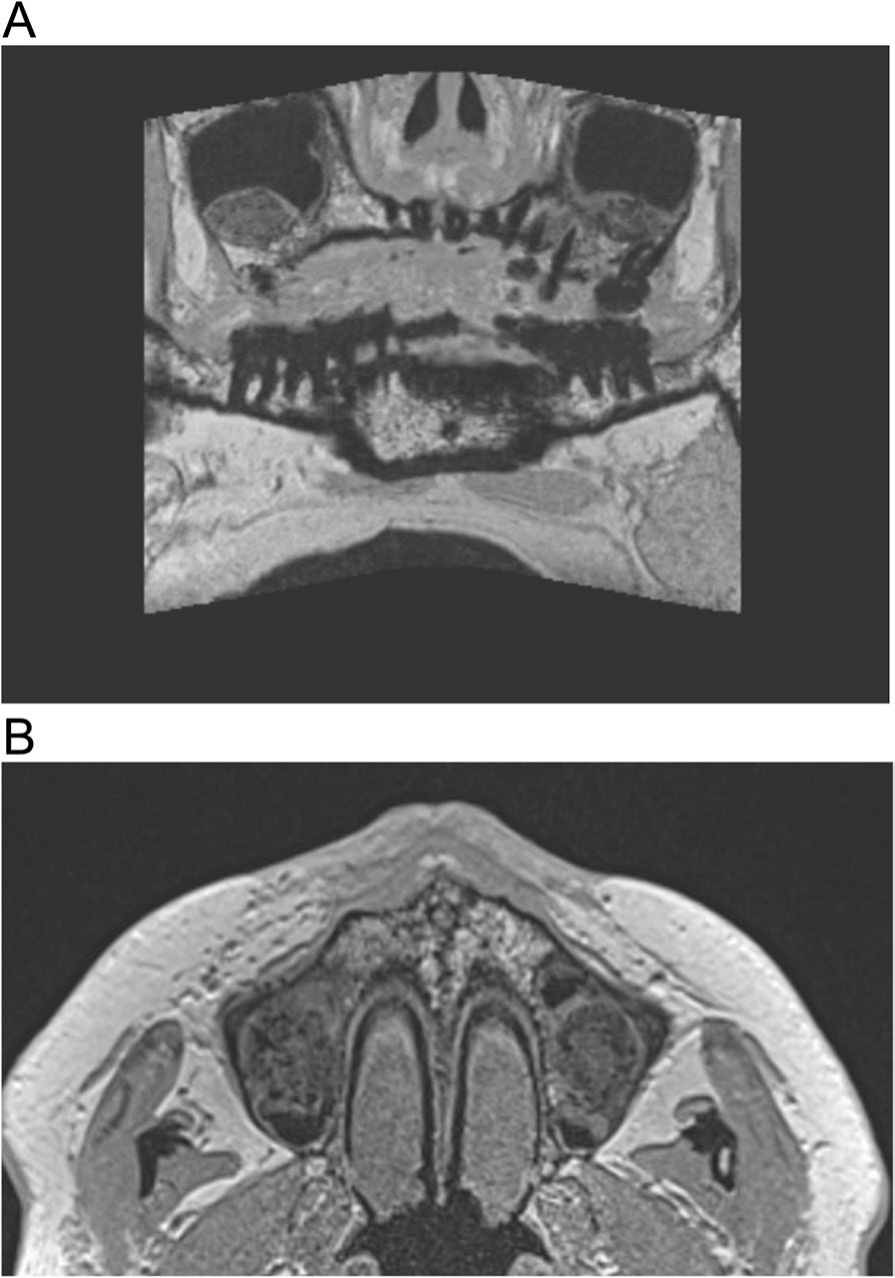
A MRI, 6 months postoperatively, coronal layer, T1 weighted. B MRI, 6 months postoperatively, transversal layer, T1 weighted sequence
